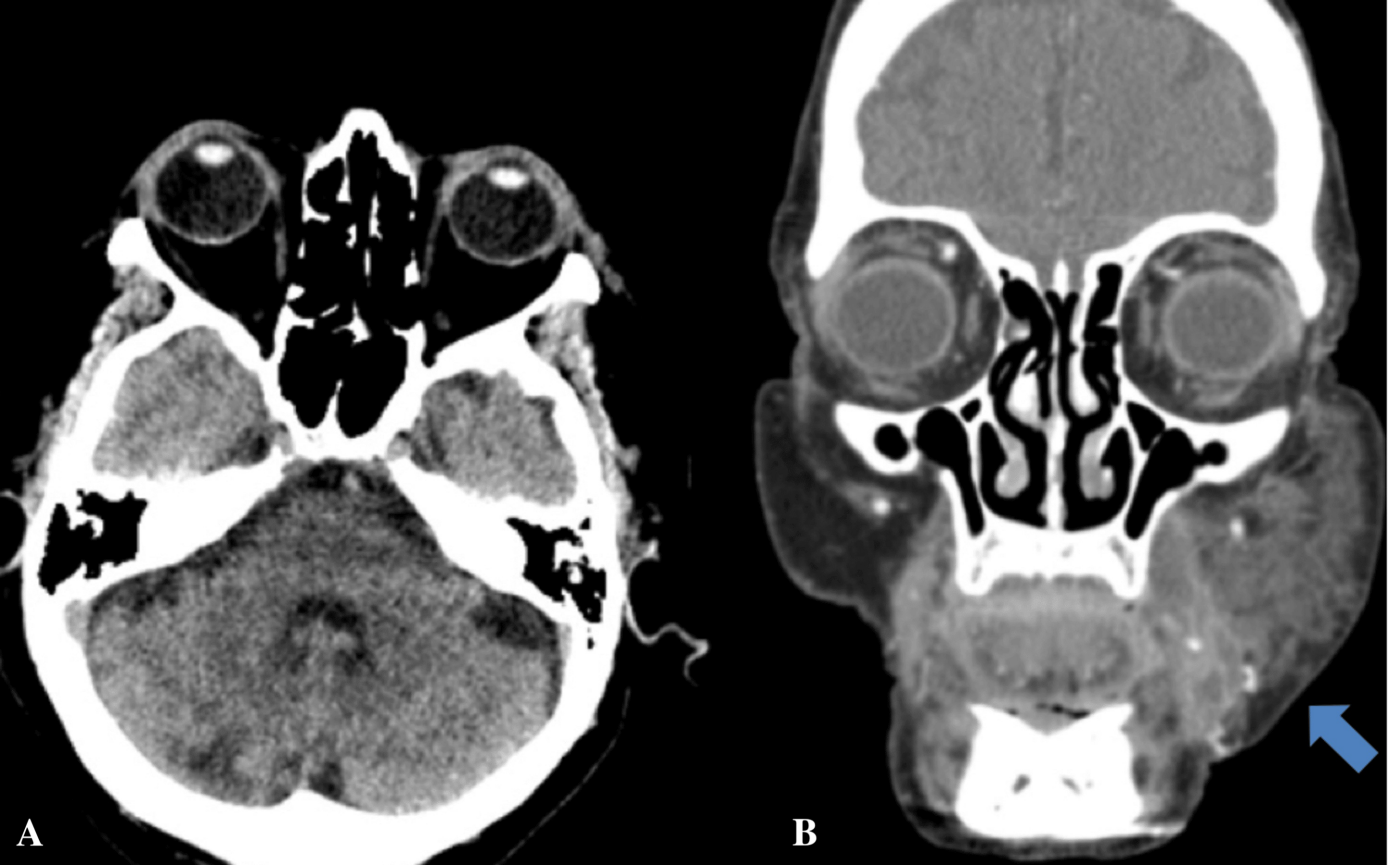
Maxillofacial CT scan - (A) axial section and (B) coronal section revealing soft tissue edema/stranding adjacent to left masticator space (blue) and unremarkable periorbital regions.
